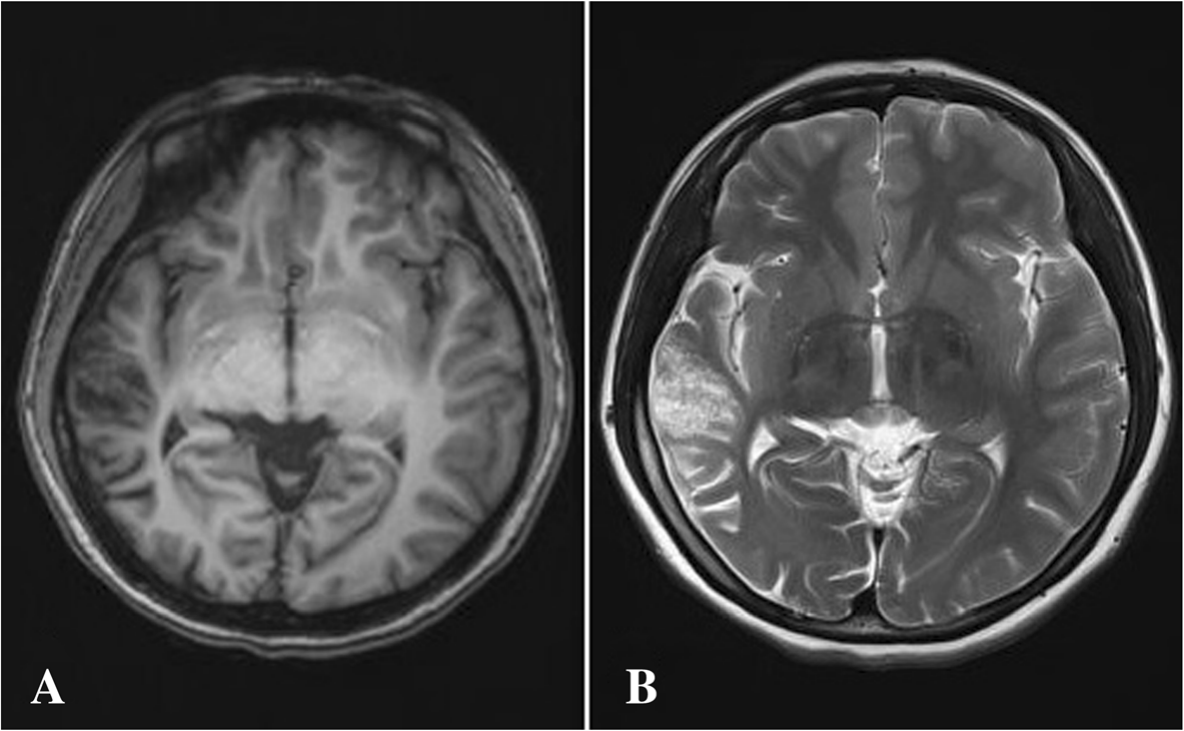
Axial brain magnetic resonance imaging (MRI) findings. A multicystic mass (arrow) with low signal intensity on T1-weighted images (A) and high signal intensity on T2-weighted images (B) is seen in the right temporal lobe. The cystic component was isointense with the cerebrospinal fluid on all sequences.
